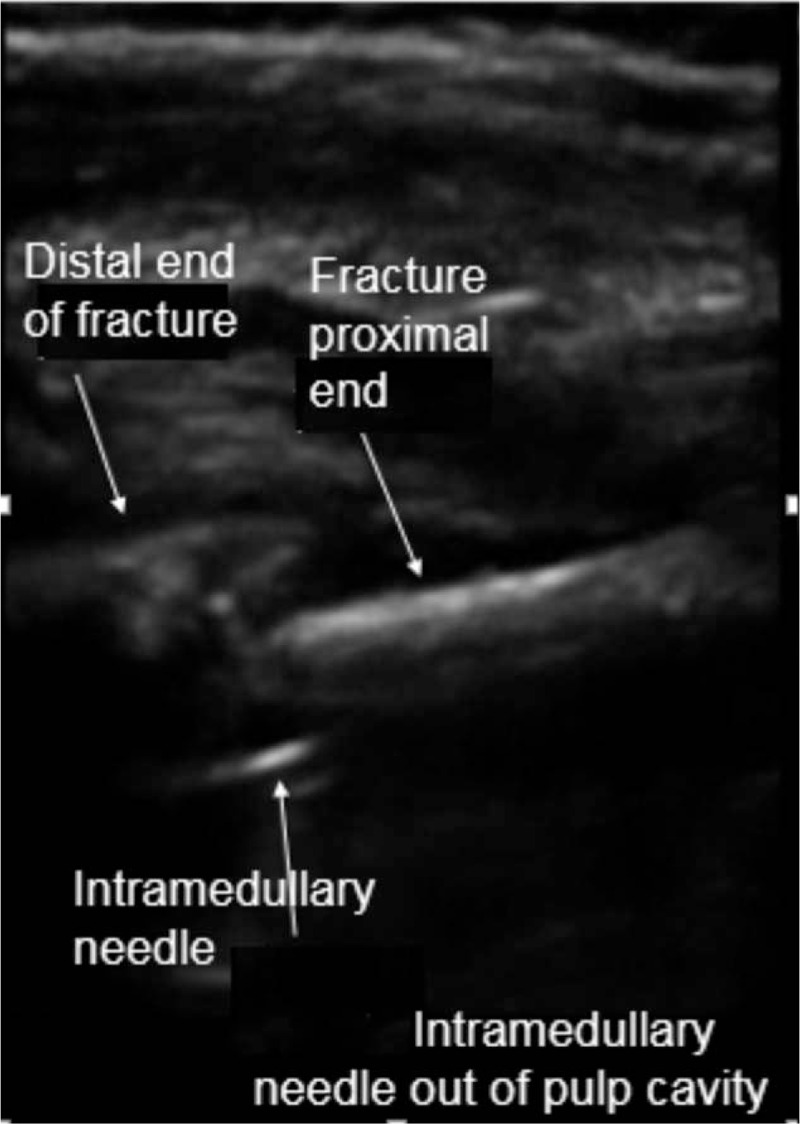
Placing nails correctly in the medullary cavity with guidance by ultrasound to detect whether the intramedullary nail exits the medullary cavity.
